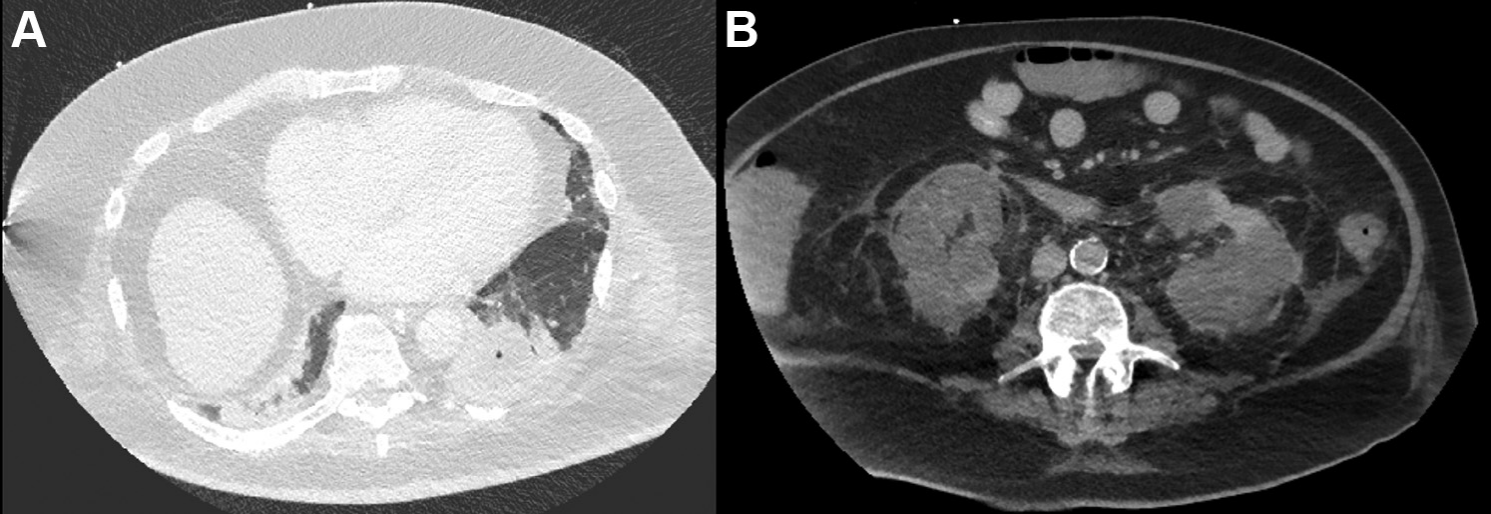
Transversal view of upper body CT scan. A, Pulmonary lesion dorsal in the left lower lobe with signs of pleural effusion and atelectasis; B, Multiple hypodense foci and edema in both kidneys.
